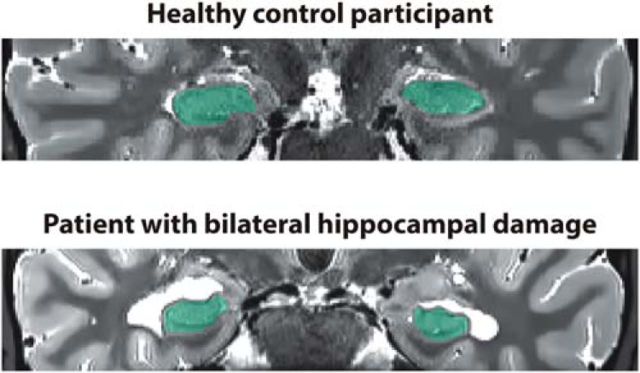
Characterization of hippocampal damage. Example T2-weighted coronal structural MR images of a healthy control participant (top) and a patient with bilateral hippocampal damage (bottom). The hippocampi are marked in green. Images are displayed in native space corresponding approximately to the position of *y* = −10 in the Montreal Neurological Institute coordinate system.
